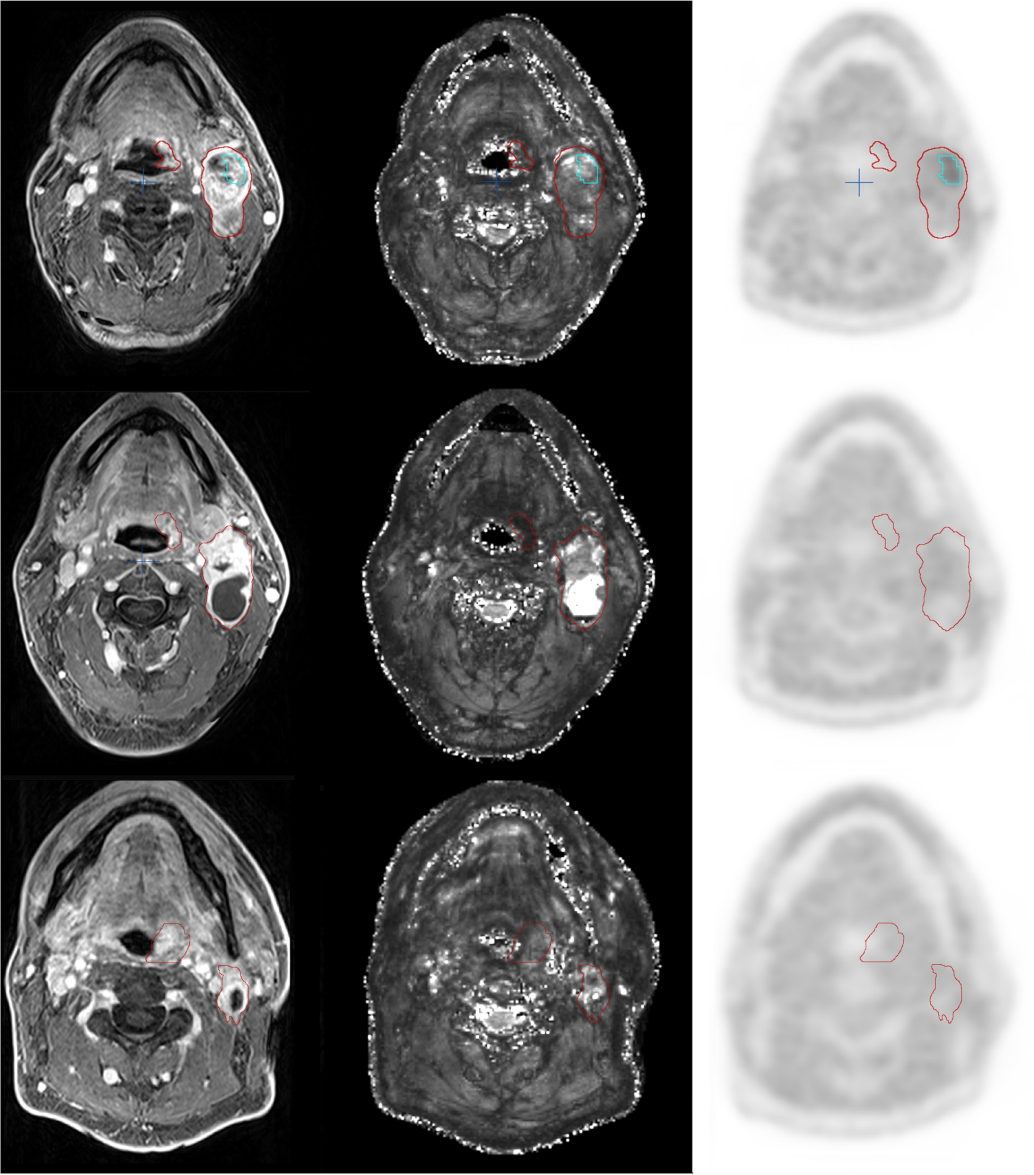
Hypoxic tumour subvolumes: T2* values* vs. *FDG uptake and FMISO uptake.* T2* values (ms) were lower and FDG uptake was higher within hypoxic tumour subvolumes as compared to non-hypoxic tumour subvolumes (p* = 0.051, *p* = 0.026). FMISO uptake was higher within hypoxic tumour subvolumes than within non-hypoxic tumour subvolumes (p* = 0.029, *p* = 0.072, *p* = 0.003, *p* = 0.0001)
